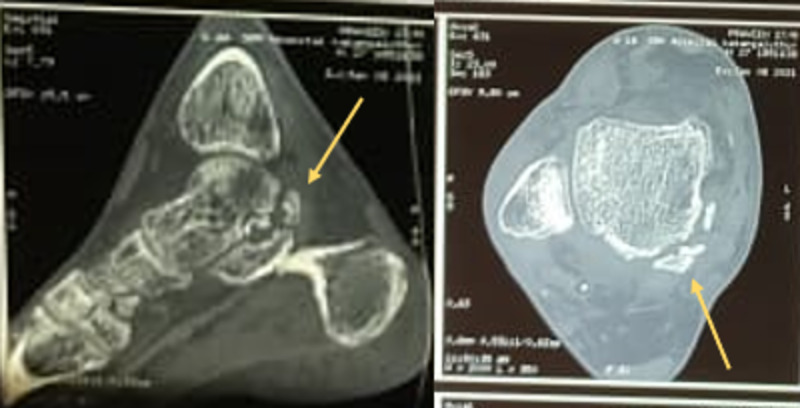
CT scan of the right ankle post-reduction showing fracture of the posterior facet of the talus.
